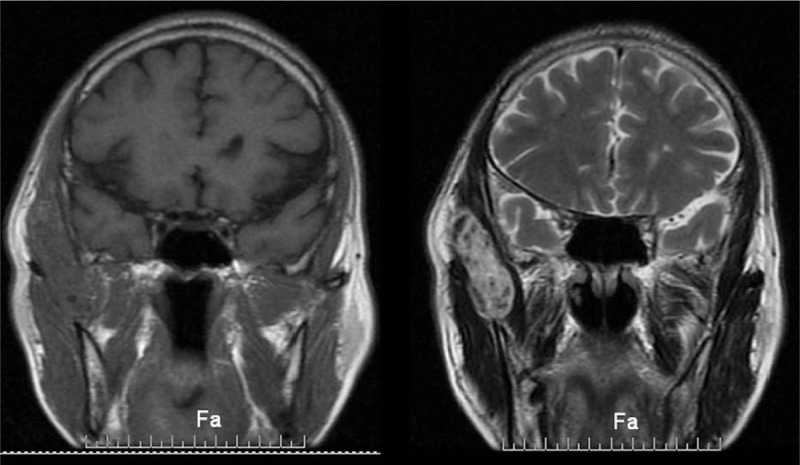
(A) T1-weighted coronal MRI scan shows a 6.2-cm ovoid mass within right temporal muscle and across the zygomatic arch. (B) T2-weighted coronal MRI scan shows millimetric hypointense structures in the mass. MRI = magnetic resonance imaging.
